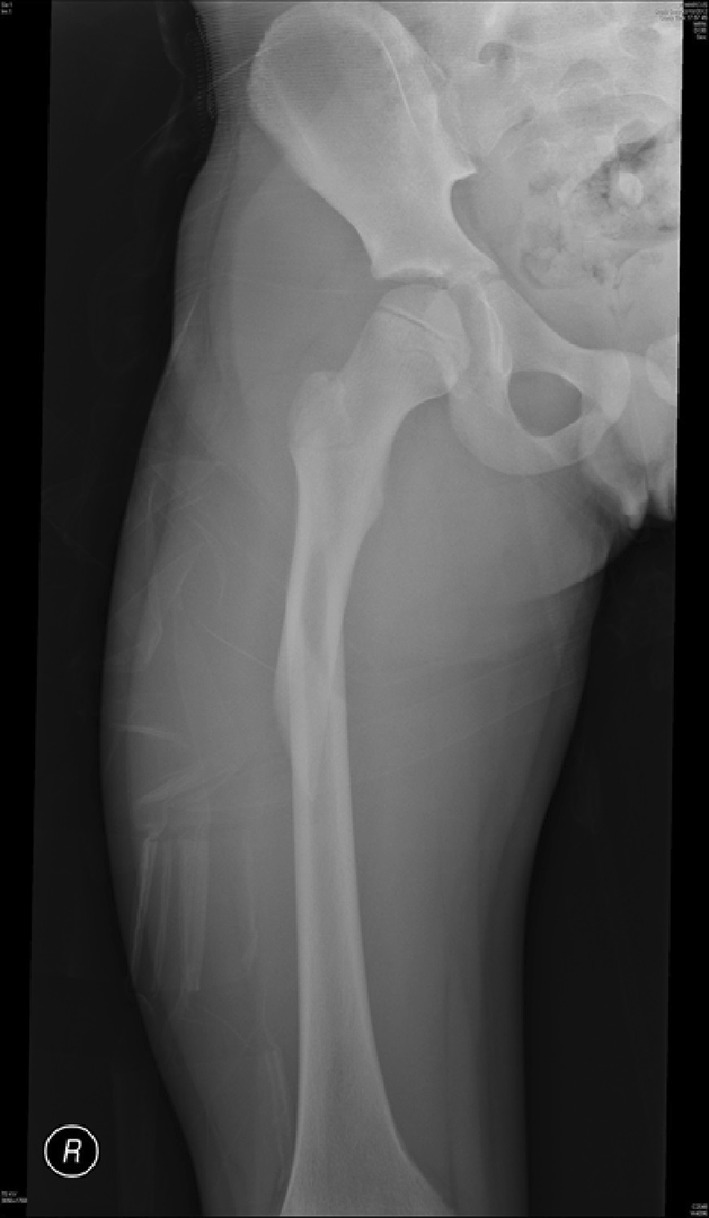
AP radiographs demonstrating a proximal 1/3 spiral femoral diaphyseal fracture in a 6-year-old child
